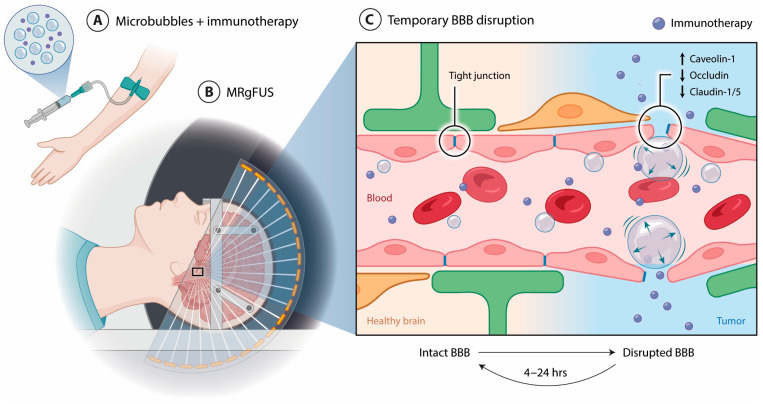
A focused ultrasound transducer is positioned over the patient’s head within the MRI patient table. (A) Microbubbles are administered intravenously along with selected immunotherapy. (B) The intracranial region is sonicated; MRI-guided focused ultrasound (MRgFUS) allows real-time image guidance and precise targeting of acoustic energy waves. (C) Upon sonication, the microbubbles interact with the ultrasonic field. At the microvascular level, microbubbles oscillate in the presence of ultrasonic waves, inducing mechanical stress on the endothelial cell wall, resulting in temporary and localized BBB disruption. This process is associated with the upregulation of caveolin-1, a key regulator in BBB permeability, and the downregulation of tight-junction proteins, including occludin, claudin-1 and -5. Collectively, this process increases BBB permeability, resolving within hours post sonication.
